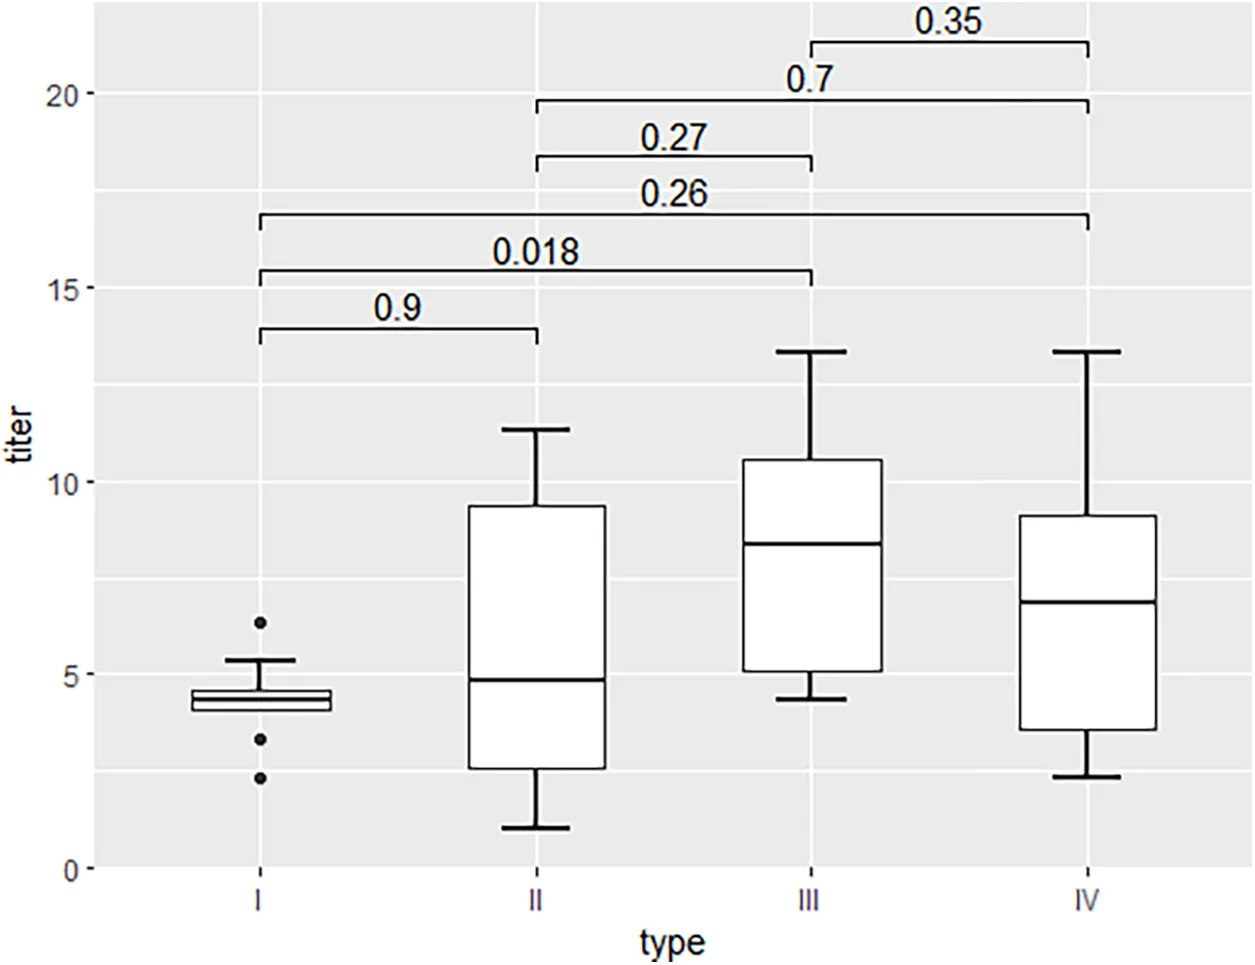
Relationship between serum cryptococcal antigen titer and CT imaging types.
